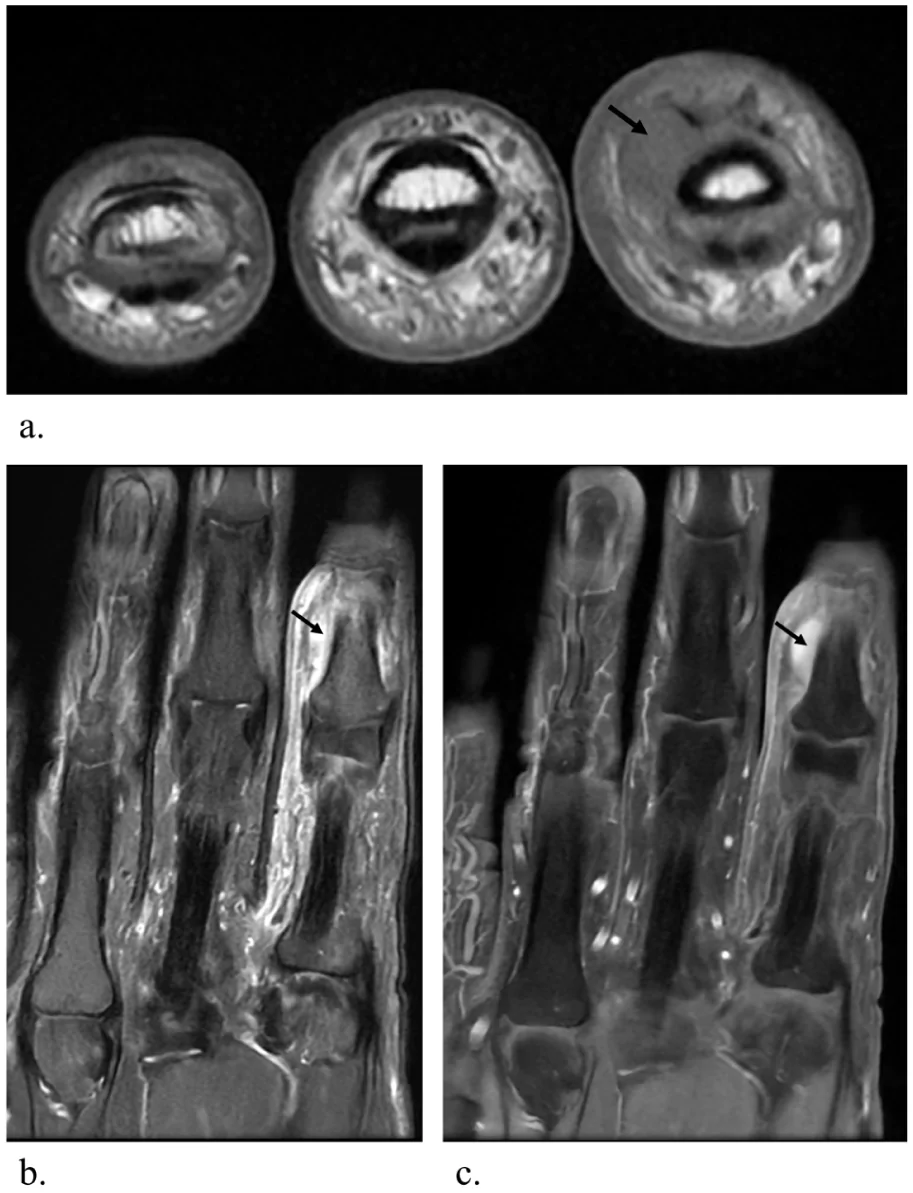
Initial presentation of the left hand on magnetic resonance imaging. (A) Axial T1: Deep subcutaneous/parosteal mass (black arrow) on the dorsal/ulnar border of the second middle phalanx, which demonstrates ill-defined low signal with infiltration of the conjoint lateral bands of the extensor mechanism. Note the absence of a T1 high signal intensity wall (penumbra sign). (B) Coronal T2 FS: The mass (black arrow) demonstrates intermediate to high signal intensity with no significant reactive marrow oedema. (C) Coronal T1 FS post-gadolinium: Uniform enhancement of the mass (black arrow) post-gadolinium administration. No medullary cavity involvement. NB: Radiographs were not initially obtained, and T2*/diffusion-weighted imaging sequences were not performed. FS indicates fat suppression.
